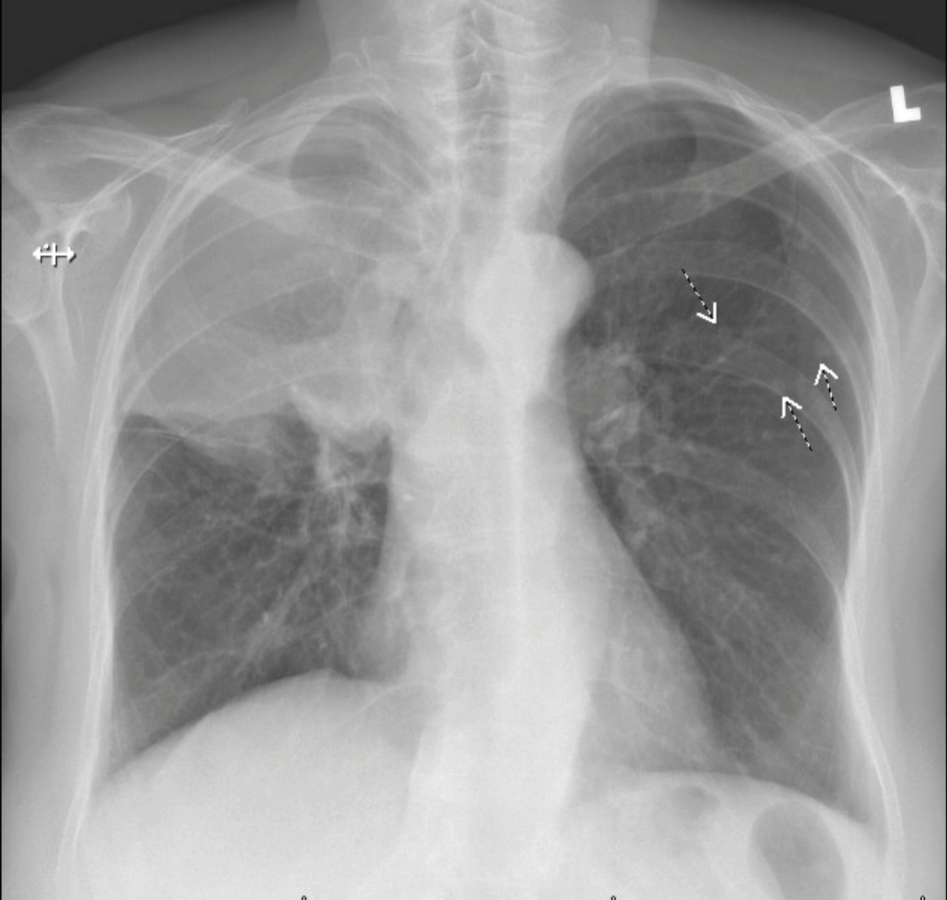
Right hilar mass/opacity with atypical right upper lung zone opacity representing mass or atelectasis. There are multiple smaller nodules in the left chest. Findings suspicious for neoplasia. CT scan of the chest with IV contrast is recommended for further evaluation.
